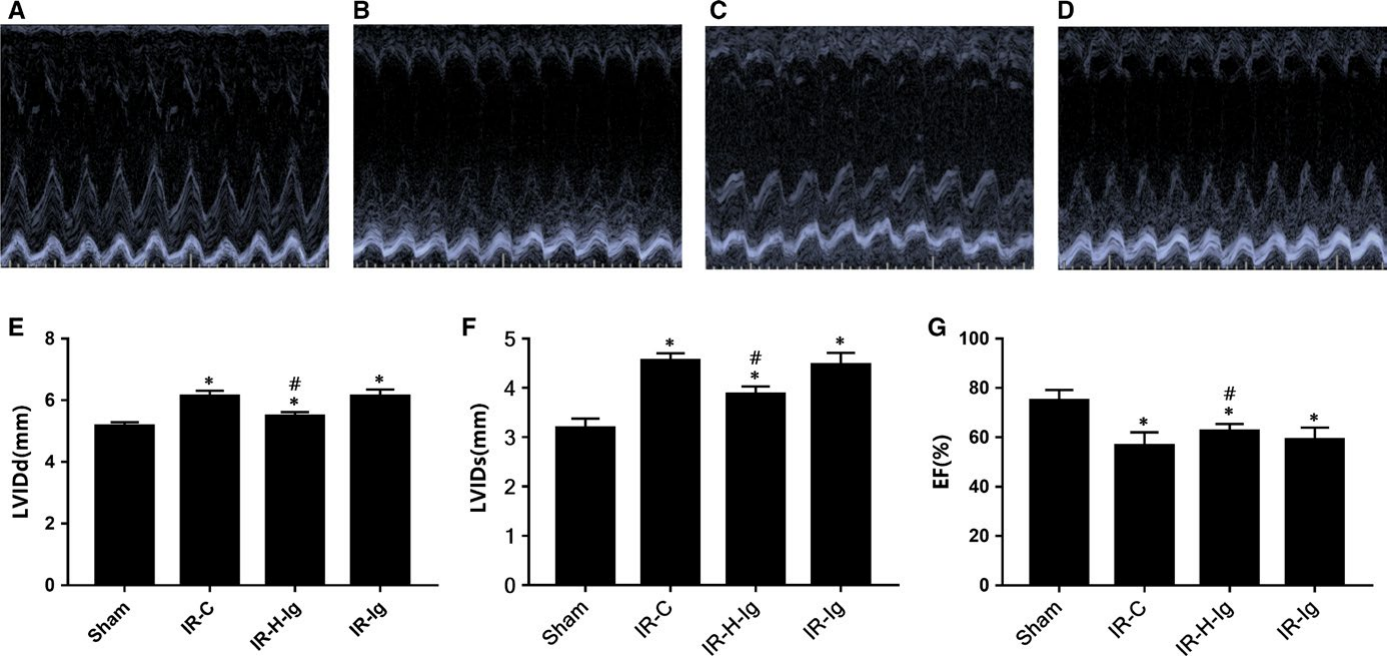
Doppler echocardiography. (A‐D) The M‐mode echocardiograms of the short‐axis midventricular view of different groups. (E‐G), The changes of left ventricular internal dimension in diastole (LVIDd), left ventricular internal dimension in systole (LVIDs) and left ventricular ejection fraction (LVEF) of different groups (n = 6 for each group). Anti‐HMGB1 treatment might improve heart function. P *< 0.05, comparisons of IR‐C, IR‐H‐Ig and IR‐Ig groups with Sham group; #*P* < 0.05, comparisons of IR‐H‐Ig and IR‐Ig group with IR‐C group
